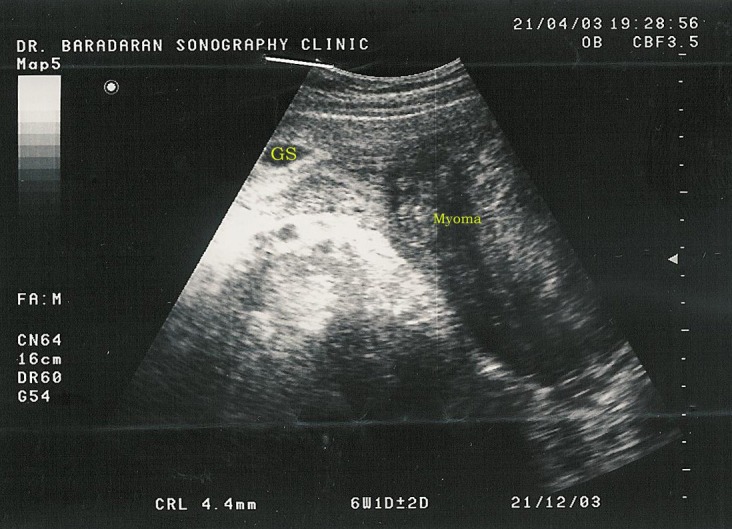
Uterine Sonography in 6 weeks of pregnancy.
